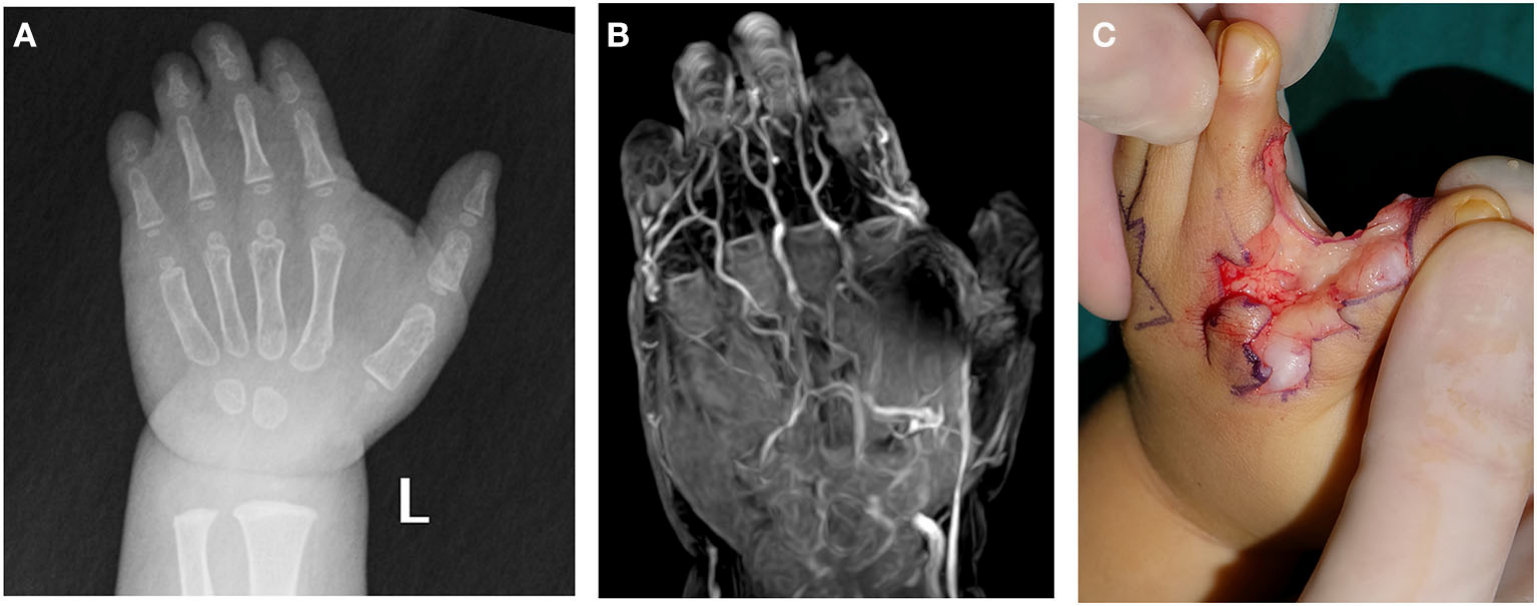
A 17-month-old female patient with complicated syndactyly of the left 2nd-3rd-4th-5th digits. (A) The preoperative radiograph showed the fused extent of soft tissues of the 2nd-3rd-4th-5th webspaces and only two phalanges of the 3rd-4th-5th digits. (B) A contrast-enhanced three-dimensional water selective cartilage scan indicated that the bifurcation positions of three common palmar digital arteries of the hand shifted distally to approximately one-third of the proximal phalanges. (C) These findings were completely consistent with the intraoperative observation results.
